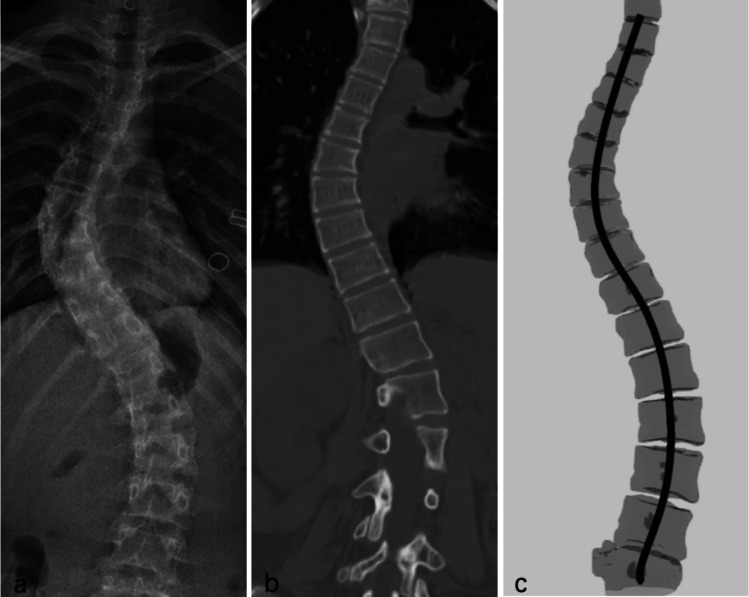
a The X-ray, b CT, and c NURBS-curve of one patient from the testing dataset
